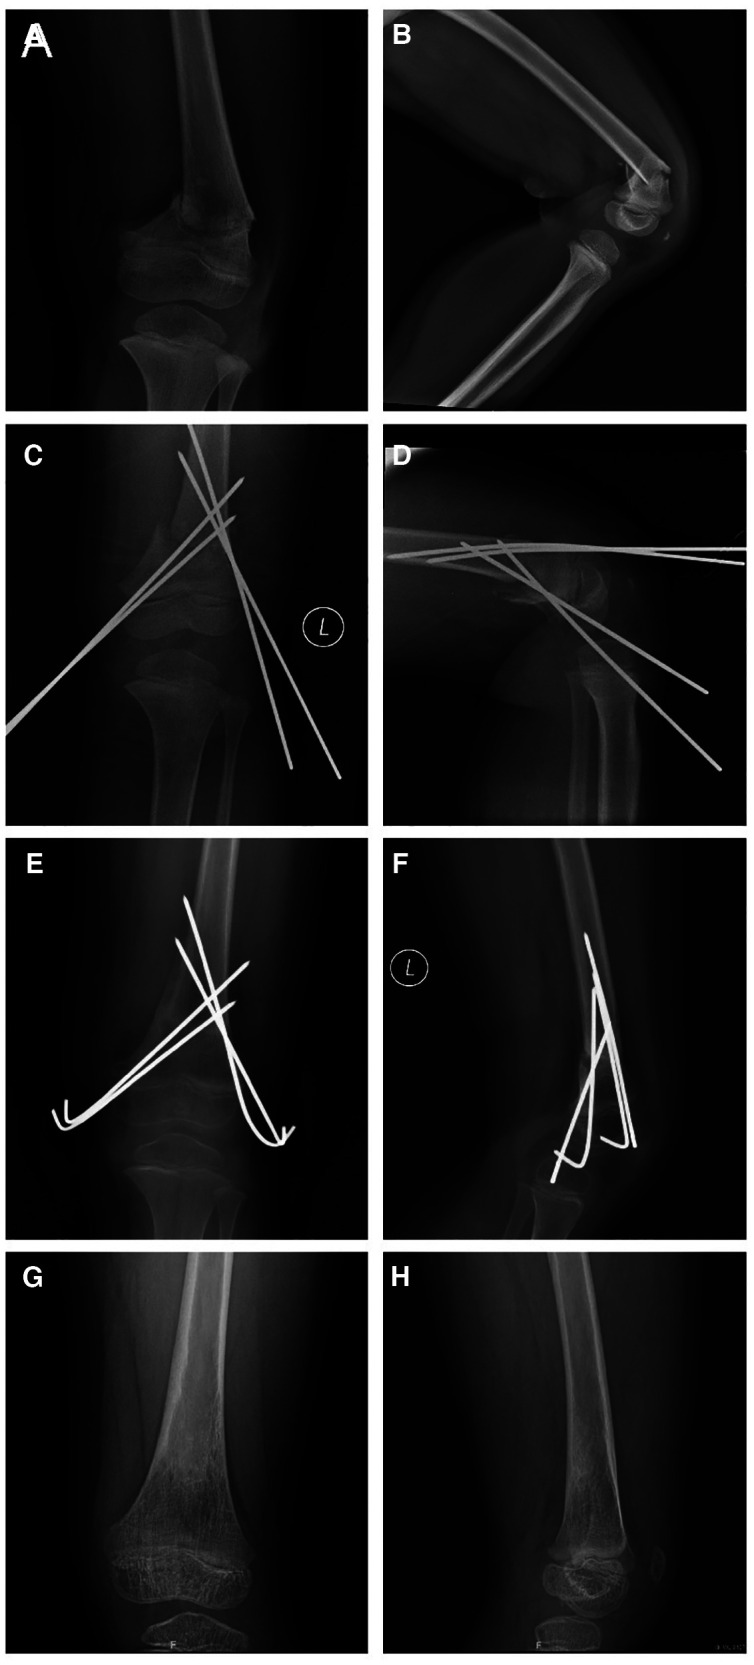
Outcome in a 4-year-old girl with a displaced supracondylar femoral fracture. Anteroposterior (A) and lateral radiographs (B) obtained before surgery. Intraoperative anteroposterior (C) and lateral radiographs (D). Anteroposterior (E) and lateral radiographs (F) obtained 1 month after surgery, and showing the fracture has healed. Anteroposterior (G) and lateral radiographs (H) obtained 1 year after surgery, which showed limb alignment was normal and epiphyseal development was normal.
